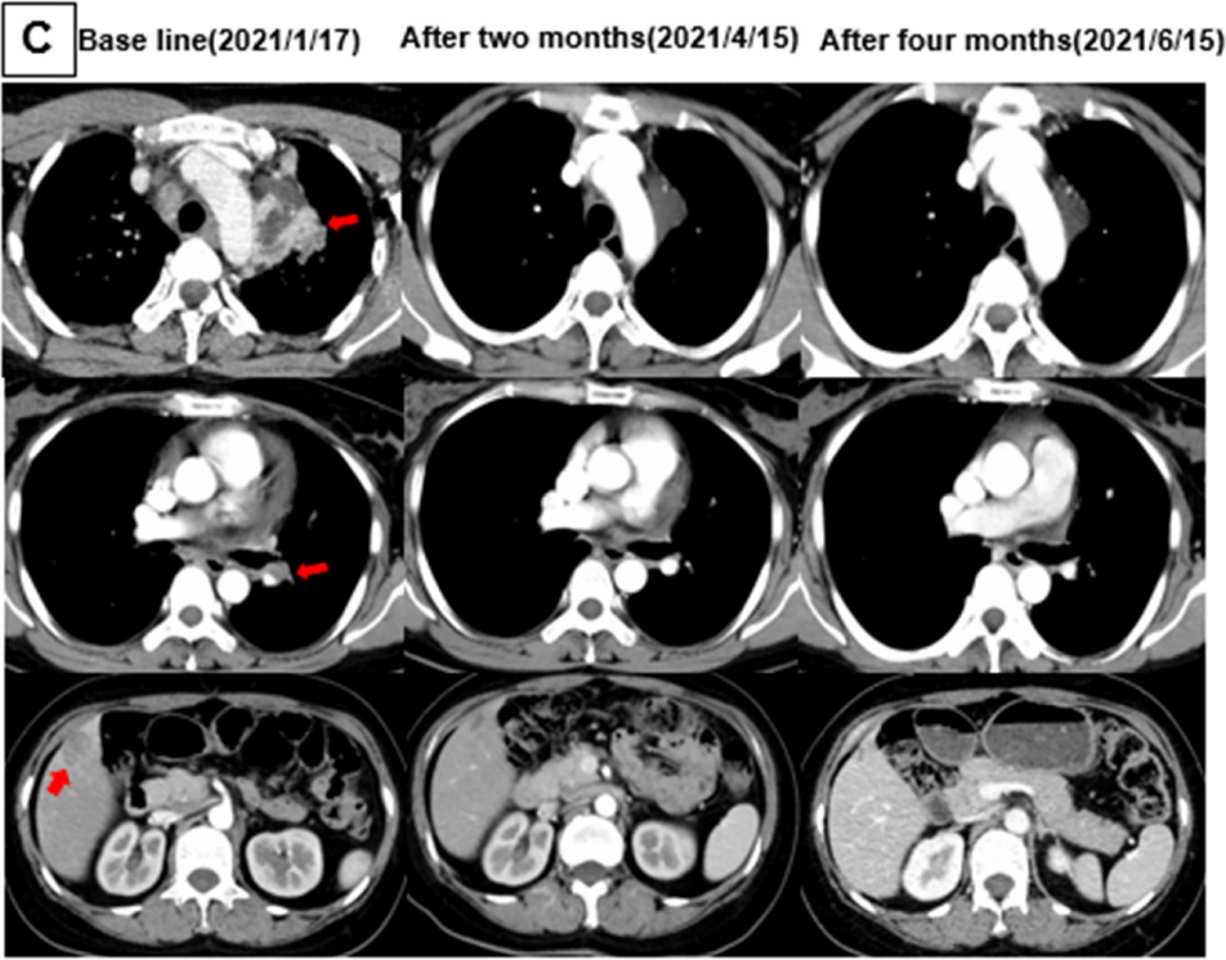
Lung adenocarcinoma shown by radiologic. Dynamic imaging of CT scan of patients treated with alectinib showing target lesions, mass invasion of the left pulmonary artery and response of liver metastases at baseline, 2 and 4 months of treatment.
